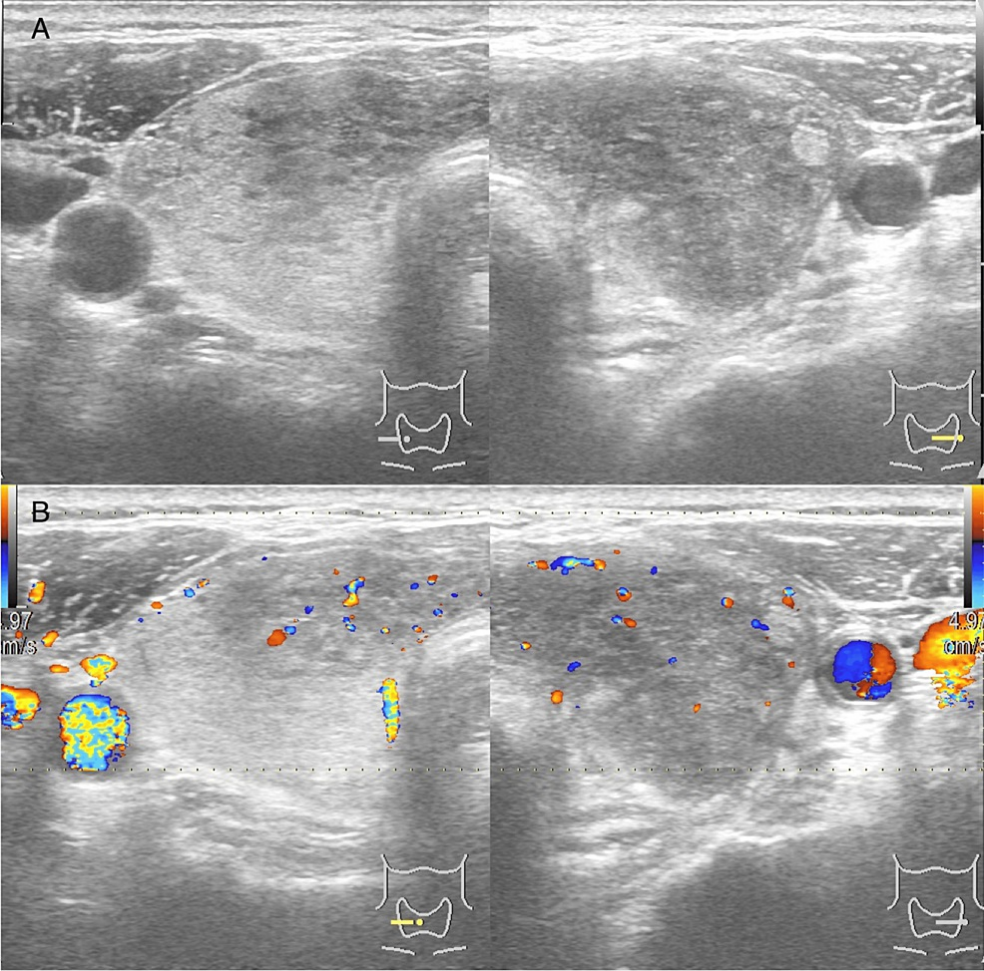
Thyroid ultrasonography and Doppler of the present case They revealed diffusely enlarged thyroid tissue with hypoechogenicity (A), and low blood flow (B).
